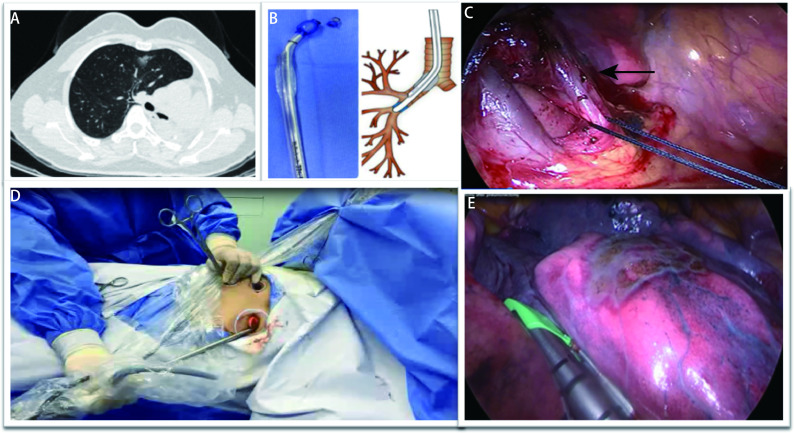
Preoperative and intraoperative figures of a patient - VATS resection of the anterior segment in the upper lobe of right lung after left pneumonectomy. A: Preoperative chest CT: Changes after left total pneumonectomy, and ground glass-like shadow in the upper lobe of right lung; B: 32-Fr left-sided double-lumen tube was used for clipping and modification to achieve a better contraposition. After anaesthesia induction, left bronchial catheter of double lumen tube was intubated into the right intermediate bronchus under the guidance of bronchoscopy to make a complete collapse in the upper lobe of right lung; C: The incision was made on both sides of the sternum; the arrow pointed to is anterior segment of right superior vein; D: A screen shot of surgical field of vision, showing the fully exposed hilum with the identification and looping of anterior segmental vein; E: Complete collapse formed in right upper lobe, with surgical staplers being applied for parenchymal transection. VATS: video-assisted thoracoscopic surgery; CT: computed tomography.
